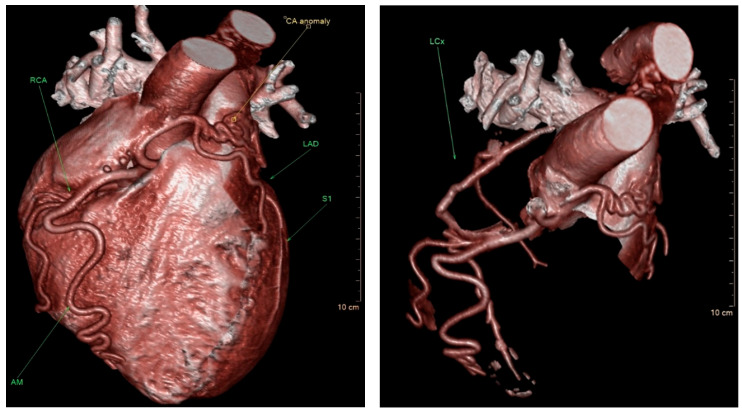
Three-dimensional volume-rendered multidetector computed tomography (MDCT) of the heart demonstrating complex coronary anomalies. Both the right coronary artery (RCA) and the left anterior descending artery (LAD) originate from the right coronary cusp (RCC). A coronary–pulmonary artery fistula is visualized between the LAD and the pulmonary artery (PA). Additionally, the left circumflex artery (LCx) is shown arising anomalously from the right branch of the PA.
